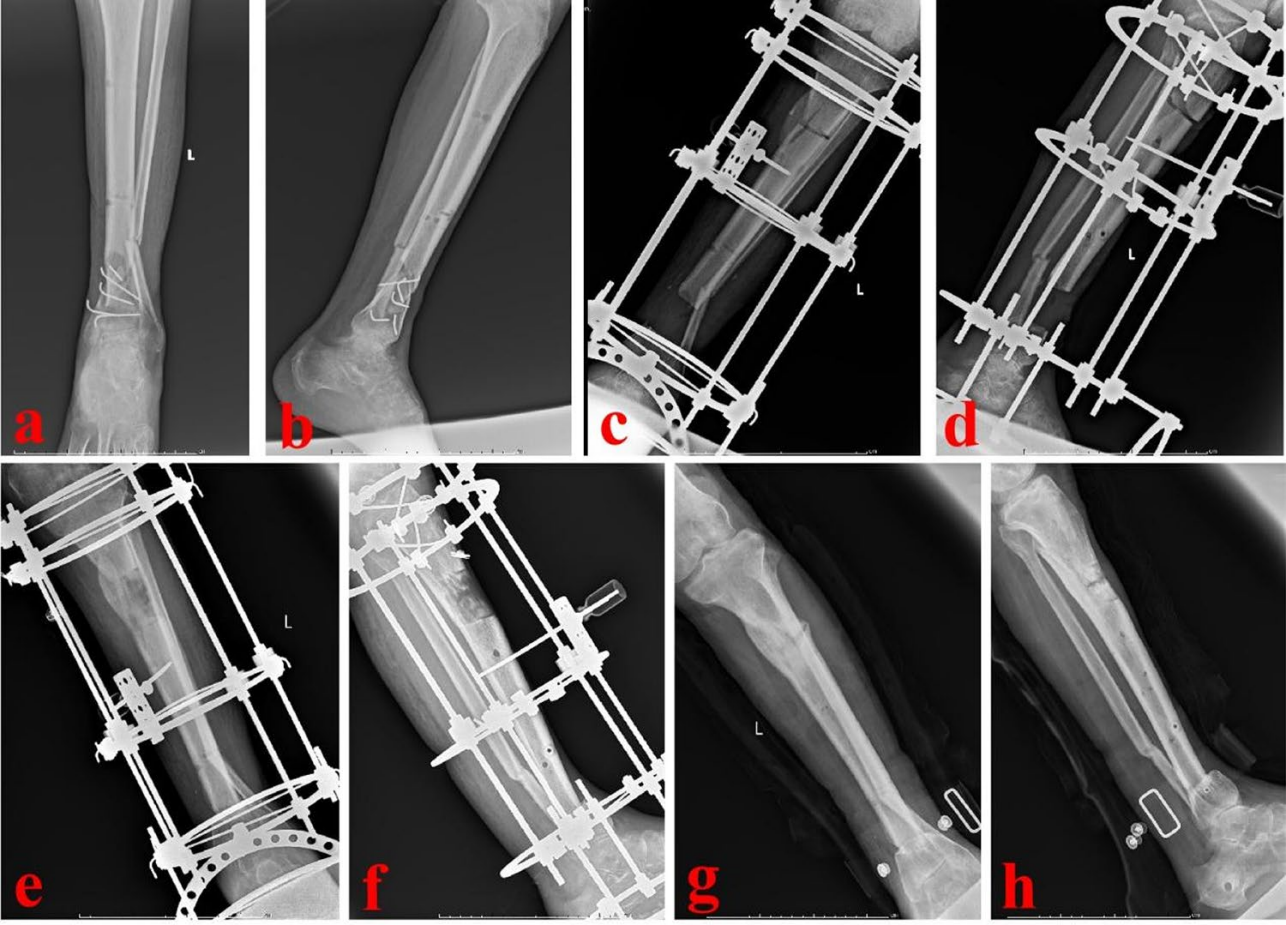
A 56-year-old male was successfully treated by the traditional bone transport technique. (a) and (b):The patient suffered a severe open fracture and infection; (c) and (d) After radical debridement, the distal tibial defects occurred. Ilizarov frame was installed and osteotomy was performed; (e) and (f) Traditional bone transport technique was successfully applied and new callus formed; (g) and (h) X-ray films after removing the Ilizarov annular frame.
